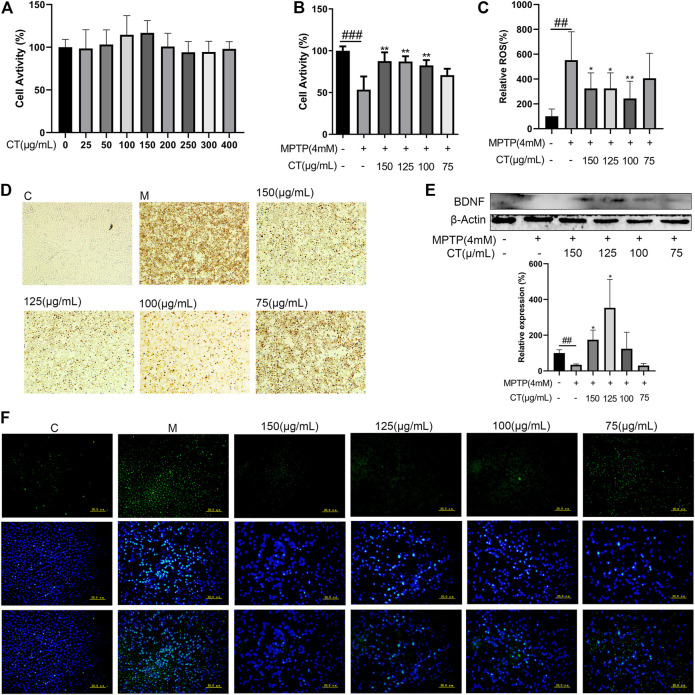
Neuroprotective effects of the CT extract. (A,B) Protective effects of CT on cell viability of SH-SY5Y without or with MPTP stimulation. (C,F) Effects of CT on intracellular ROS level. (D) Effects of CT on cell apoptosis by TUNEL assay. (E) Effects of CT on protein expression level of BDNF in MPTP-induced SH-SY5Y cells. Data were shown as mean ± SD (*n* = 3). ^#^
